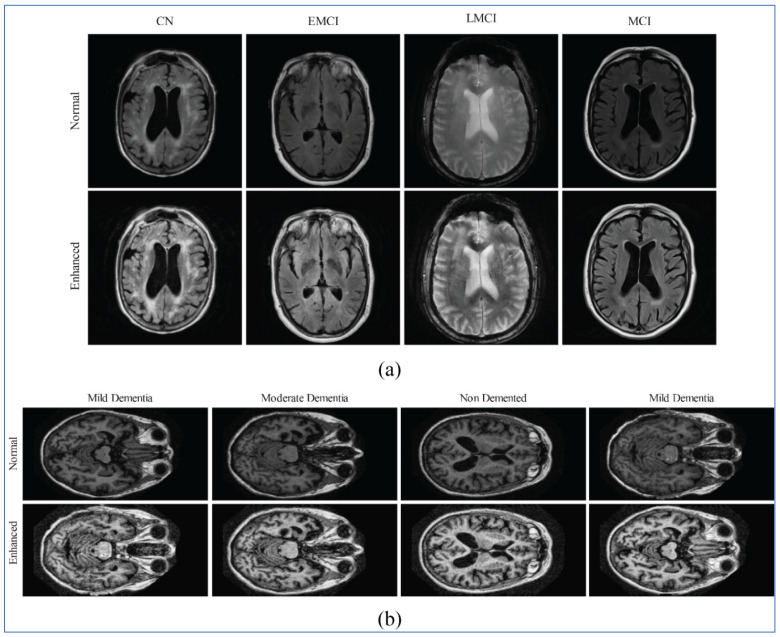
Sample MRI images from the ADNI and OASIS datasets with their corresponding contrast-enhanced versions. Subfigure (a) shows examples from the ADNI dataset, while (b) presents samples from the OASIS dataset. The contrast enhancement step improves the visibility of anatomical structures, facilitating better feature extraction for Alzheimer’s disease prediction.
